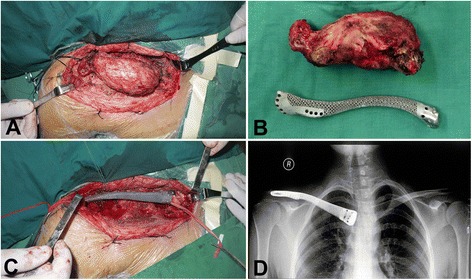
The clavicle tumor resection and prosthetic reconstruction. a Exposure of Ewing’s sarcoma. b The size of excised tumor and prosthesis was well matched. c Implantation and fixation of prosthesis. d X-ray showed proper placement and clavicular symmetry at 24 months postoperatively
